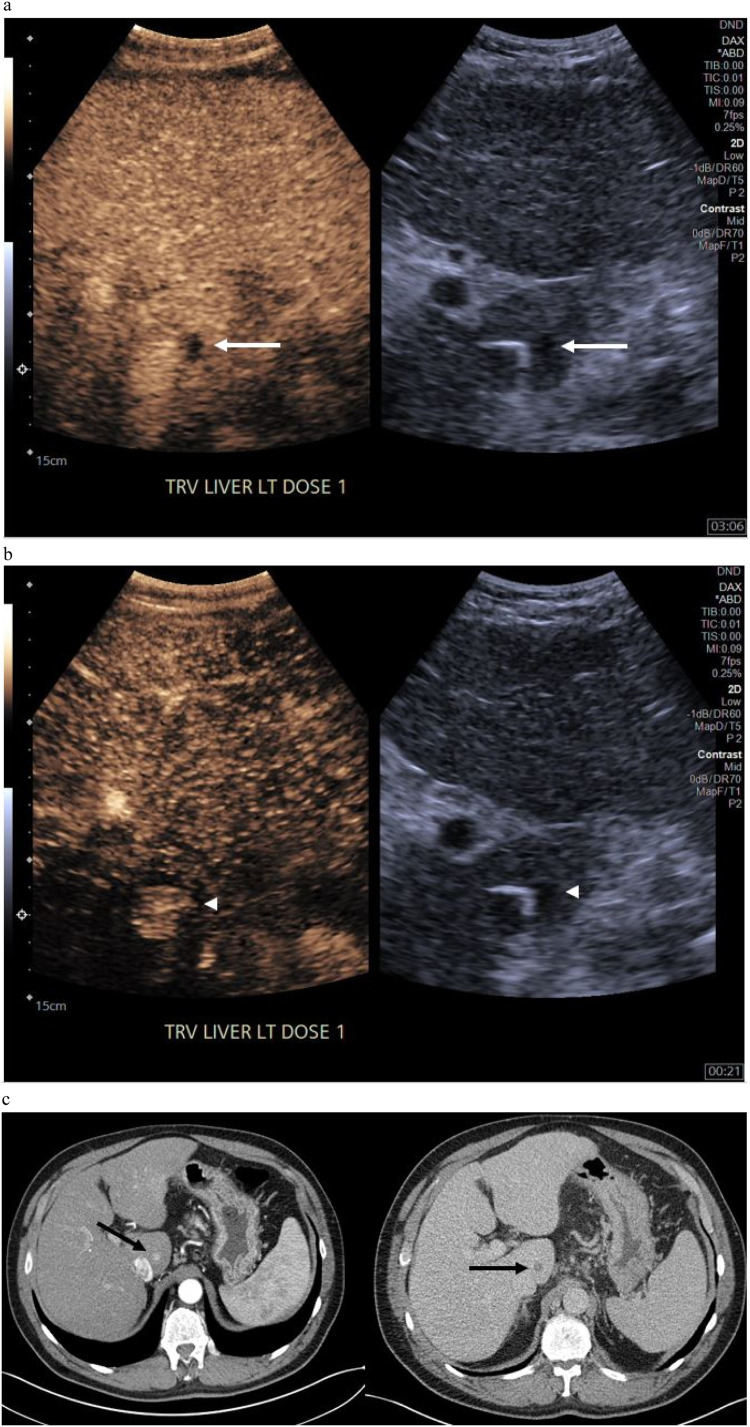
LIRADS-M OAC lesion – identified only after contrast administration and first recognized on delayed phase (a) white arrow. Arterial phase, the lesion was seen in retrospect when searching cine clips specifically for it (b), white arrow head. (c) Arterial and delayed phases show corresponding lesion on CT, black arrows.
